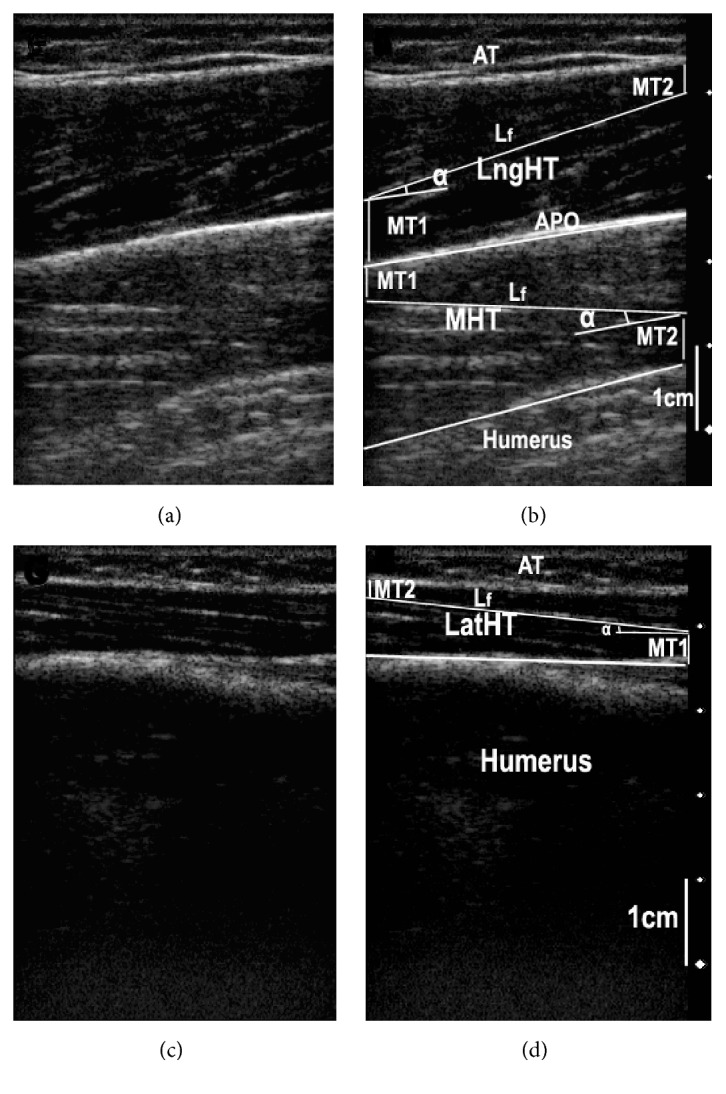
Typical ultrasonography imaging of the prime elbow extensors: long head of triceps brachii (LngHT), medial head of triceps brachii (MHT), and lateral head of triceps brachii muscle. (a) Original ultrasound image of MHT and LngHT; (b) MHT and LngHT image with labels; (c) original ultrasound image of LatHT; and (d) LatHT image with labels. In (a) and (b), the APO (bright fringe in the middle region) shows the boundary between MHT and LngHT. The white fringe in the lower range is the muscle-bone boundary (MHT-humerus). In (c) and (d), the bright fringe shows the muscle-bone boundary (LatHT-humerus). In (b) and (d), *L*
